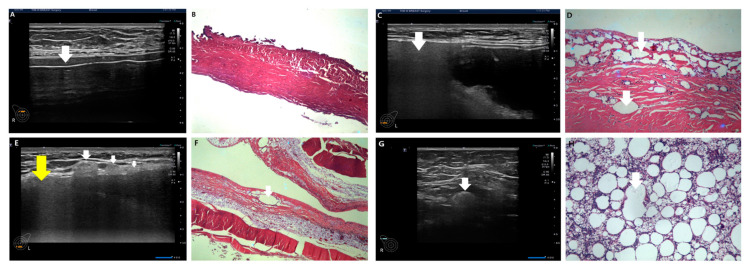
Ultrasonographic images of silicone breast implant rupture. Comparison between ultrasonographic findings regarding pathology. (A) Subcapsular silicone rupture in ultrasonography (a white arrow indicates free silicone); (B) pathologic finding of subcapsular rupture (there is no silicone migration in capsule); (C) intracapsular silicone rupture in ultrasonography (a white arrow indicates free silicone); (D) pathologic finding of intracapsular silicone invasion (a white arrow indicates silicone migration); (E) extracapsular silicone migration to adjacent tissue with snowstorm sign (snowstorm sign indicated by a yellow arrow, silicone migration to adjacent tissue is indicated by a white arrow); (F) pathologic finding of extracapsular silicone migration (a white arrow characterizes silicone migration to capsules); (G) silicone migration to regional lymph nodes (a white arrow indicates snowstorm artifact); and (H) pathologic finding of silicone migration to a regional lymph node(a white arrow indicates free silicone).
